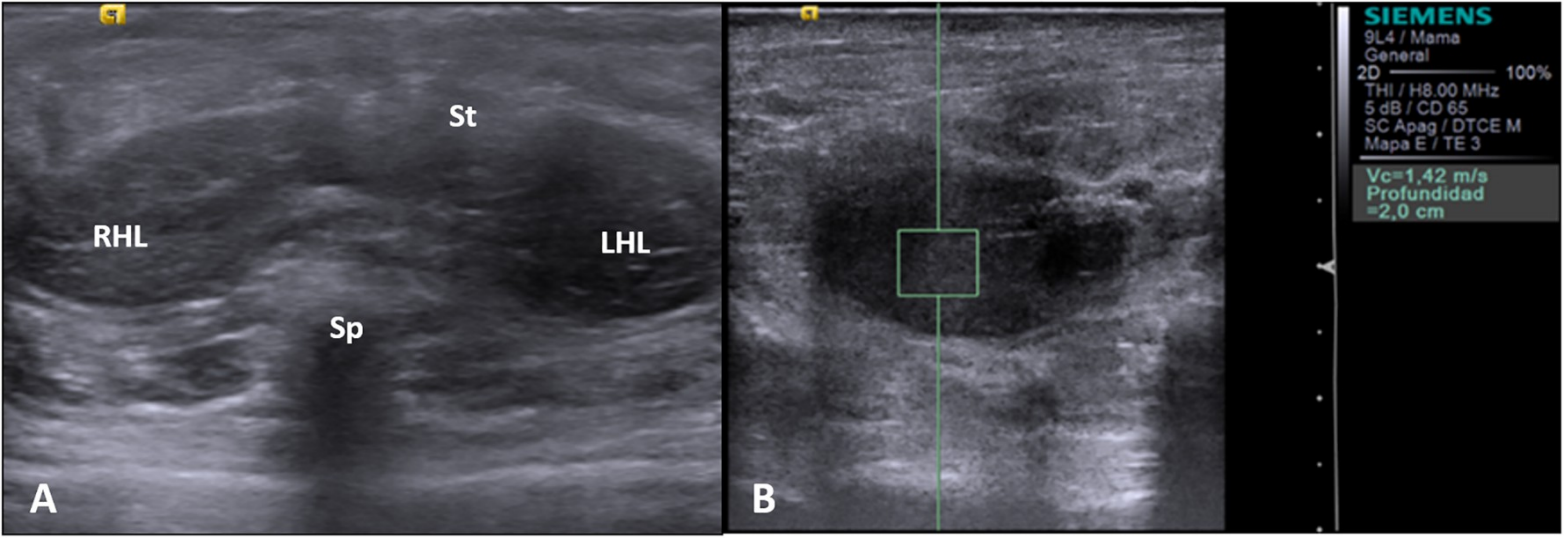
A, *In vivo* ultrasound image of the upper abdominal quadrants. RHL: right hepatic lobe; LHL: left hepatic lobe; St: Stomach; Sp: Spine. B, *In vivo* assessment using the ARFI technique.
